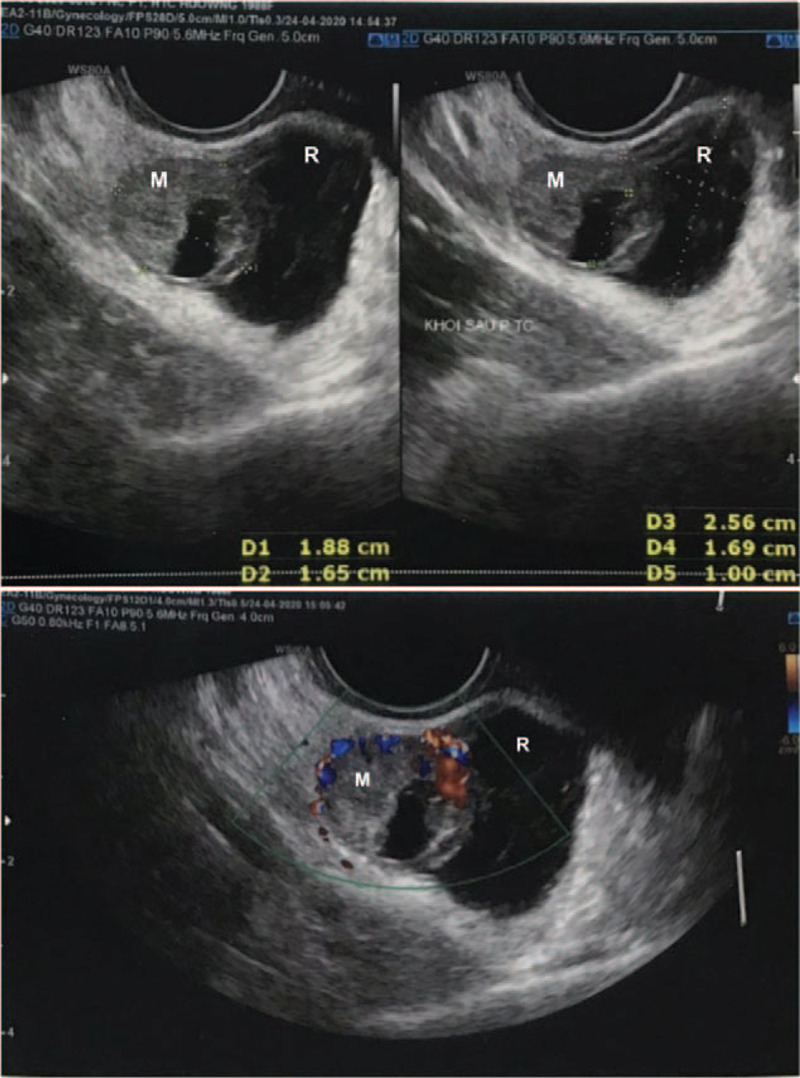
Transvaginal Doppler ultrasound showing a 19 × 17 mm mass with a yolk sac on the day of admission (M: mass, R: rectum).
